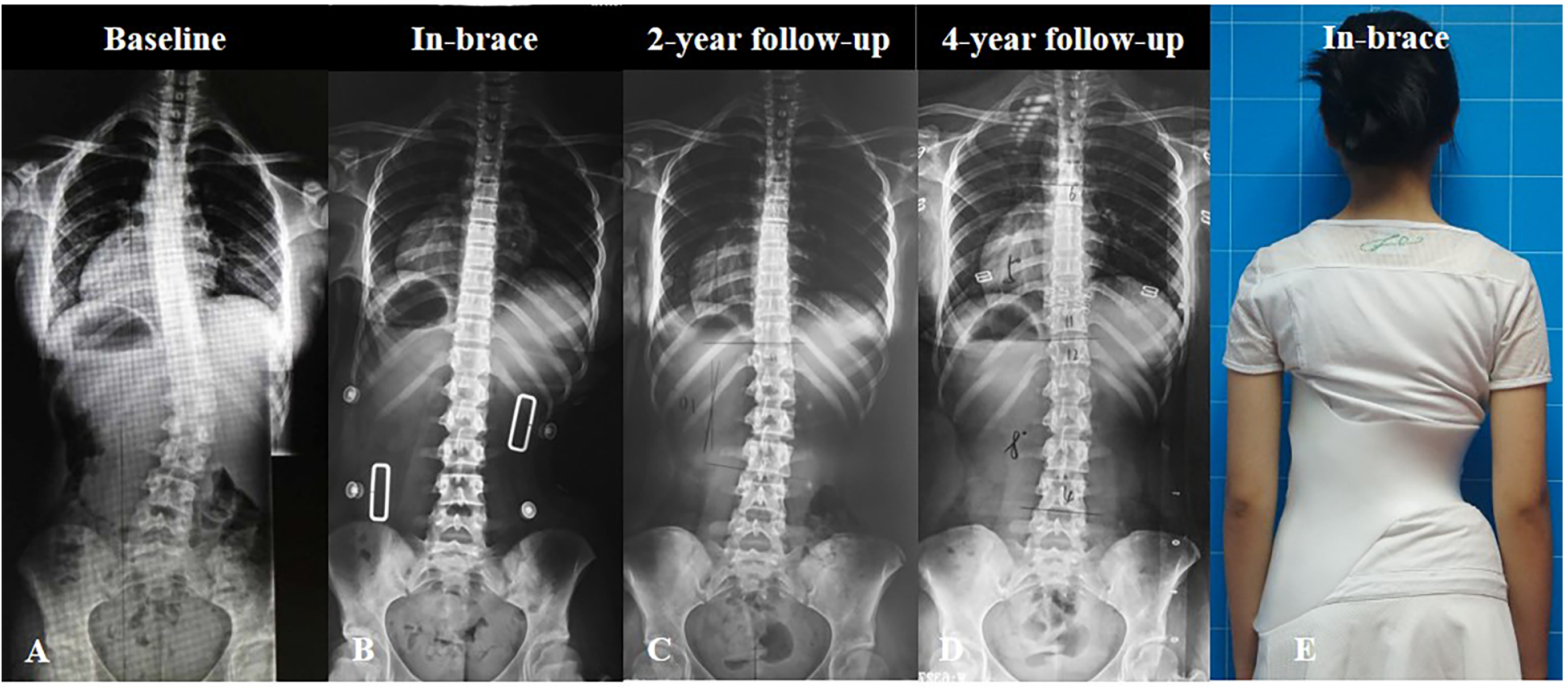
A 12-year-old girl diagnosed with adolescent idiopathic scoliosis was treated with lumbosacral orthosis (LSO). The main lumbar curve was 30° and the minor thoracic curve was 18° at baseline (A) The in-brace radiograph showed excellent correction of both curves (B) After 2 years of treatment, the patient had satisfactory curve improvement (C), which was adequately maintained over a 4-year follow-up period (D) Clinical in-brace appearance of the patient (E).
